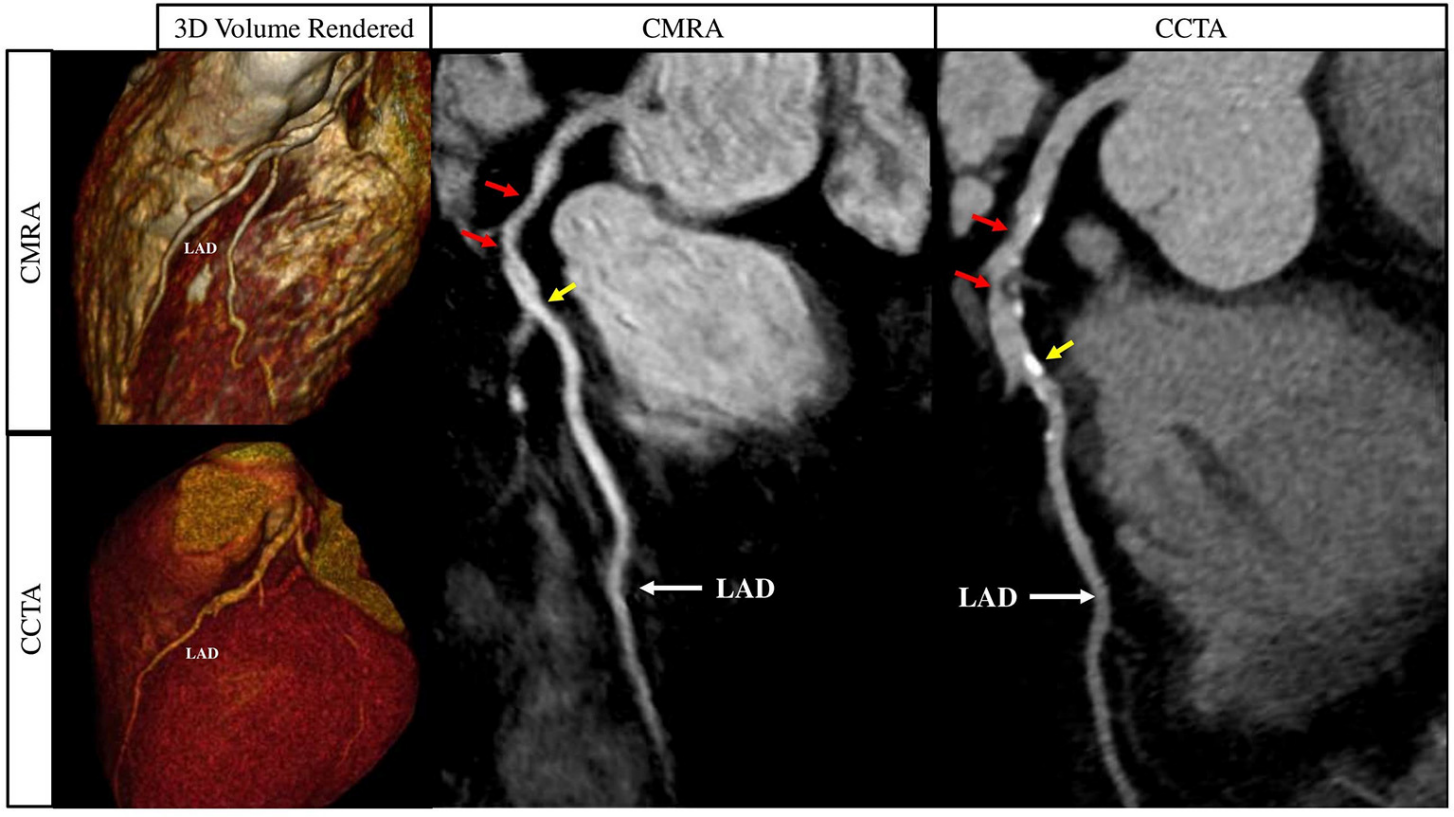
Curved multiplanar reformat and 3D volume rendered non-contrast CMRA and contrast enhanced CCTA in a 60 year old male with >50% partially calcified stenosis in the proximal to mid LAD on either side of the first diagonal artery (red arrows). The yellow arrows represent a focal calcified <50% stenosis just distal to the second diagonal artery. CMRA, Coronary Magnetic Resonance Angiography; CCTA, Coronary Computed Tomography Angiography; LAD, Left Anterior Descending Artery. Adapted with permission from Hajhosseiny et al. (66).
